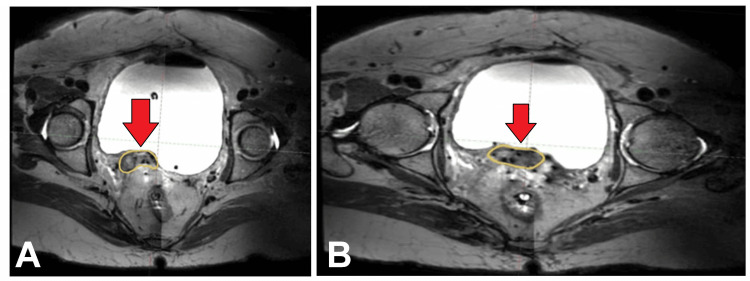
(A,B) Images of the T2-weighted MRI showing the residual disease in the vault of the patient after completing external beam radiotherapy (red arrows) MRI: magnetic resonance imaging
